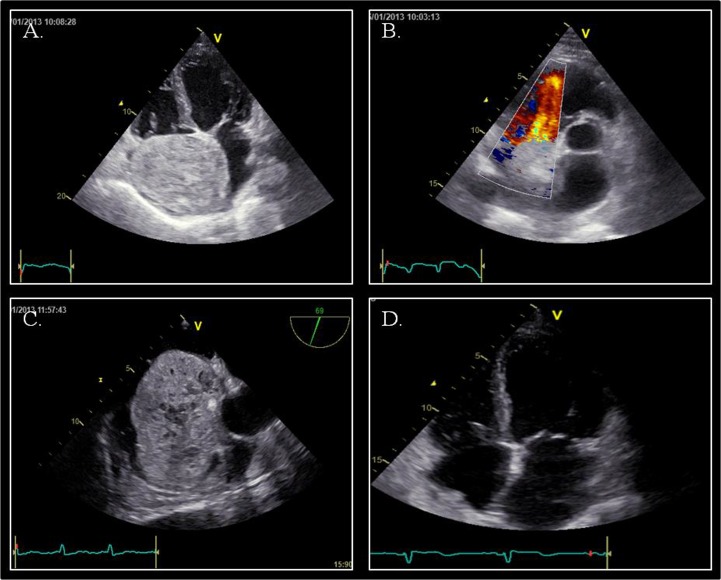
Echocardiography Panel A. Apical four-chamber view showing a voluminous mass adhering to inter-atrial septum. Panel B. Parasternal short-axis view: turbulence in the right ventricular inflow tract; no signs of right outflow obstruction. Panel C. Mid-oesophageal aortic valve long axis view (70°): atrial mass with evidence of parenchymatous density and multiple echolucent structures occupying almost the whole right atrium with no evidence of caval veins obstruction. Panel D. Apical four-chamber view after surgical removal of the tumor mass.
